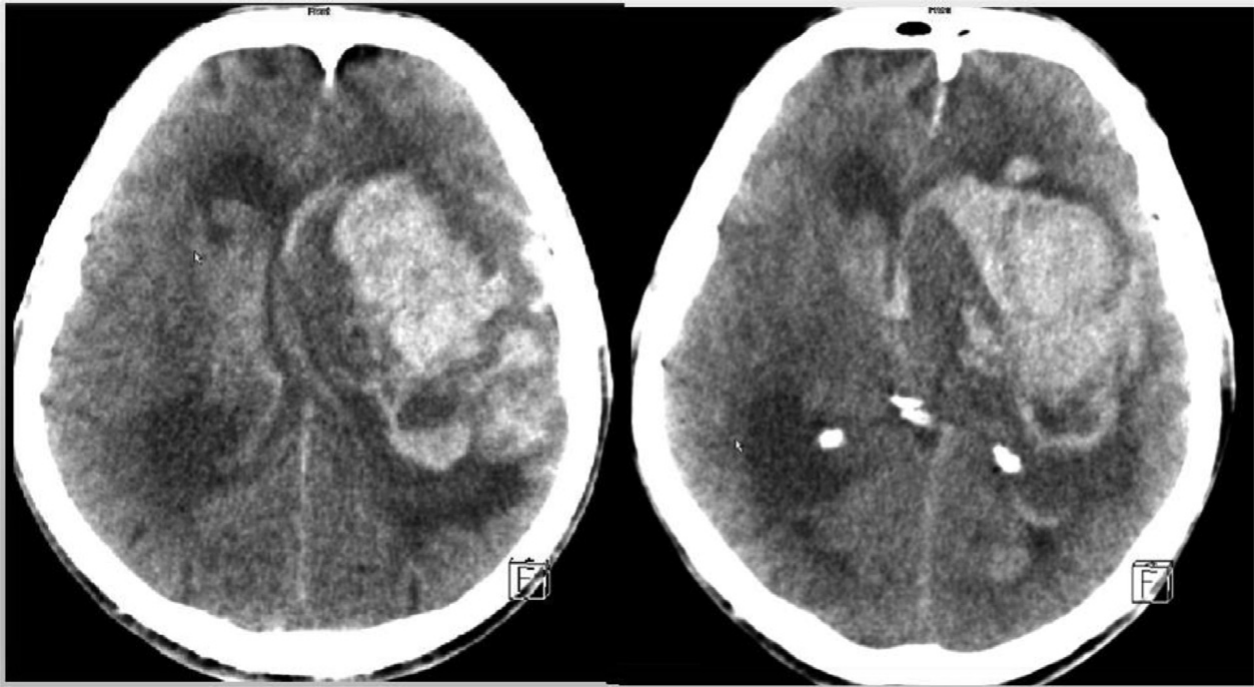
Cerebral CT scan showing intra-axial mass bleeding with ventricular rupture in a left fronto-temporal distribution.
